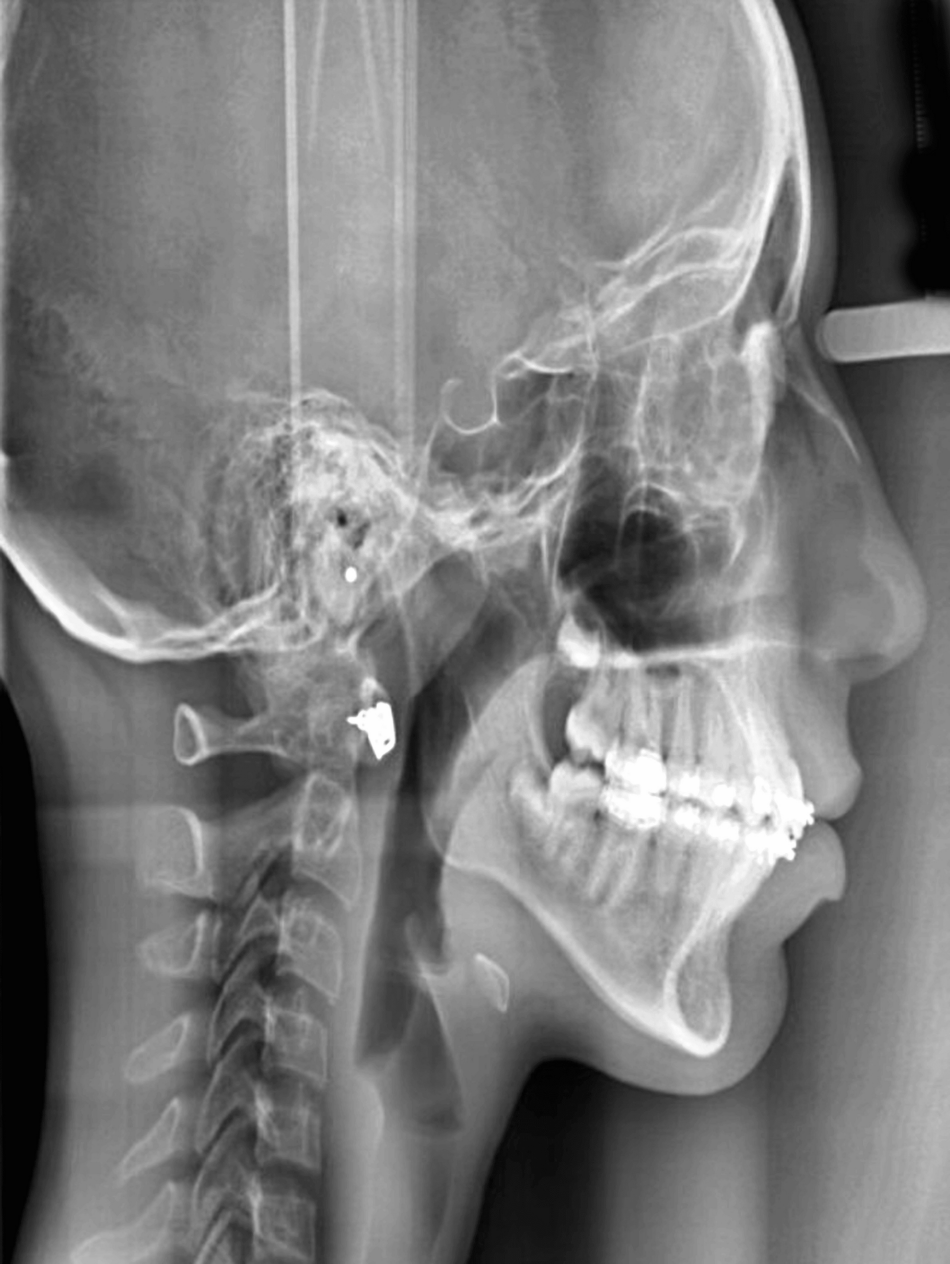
Post-treatment orthopantomogram
